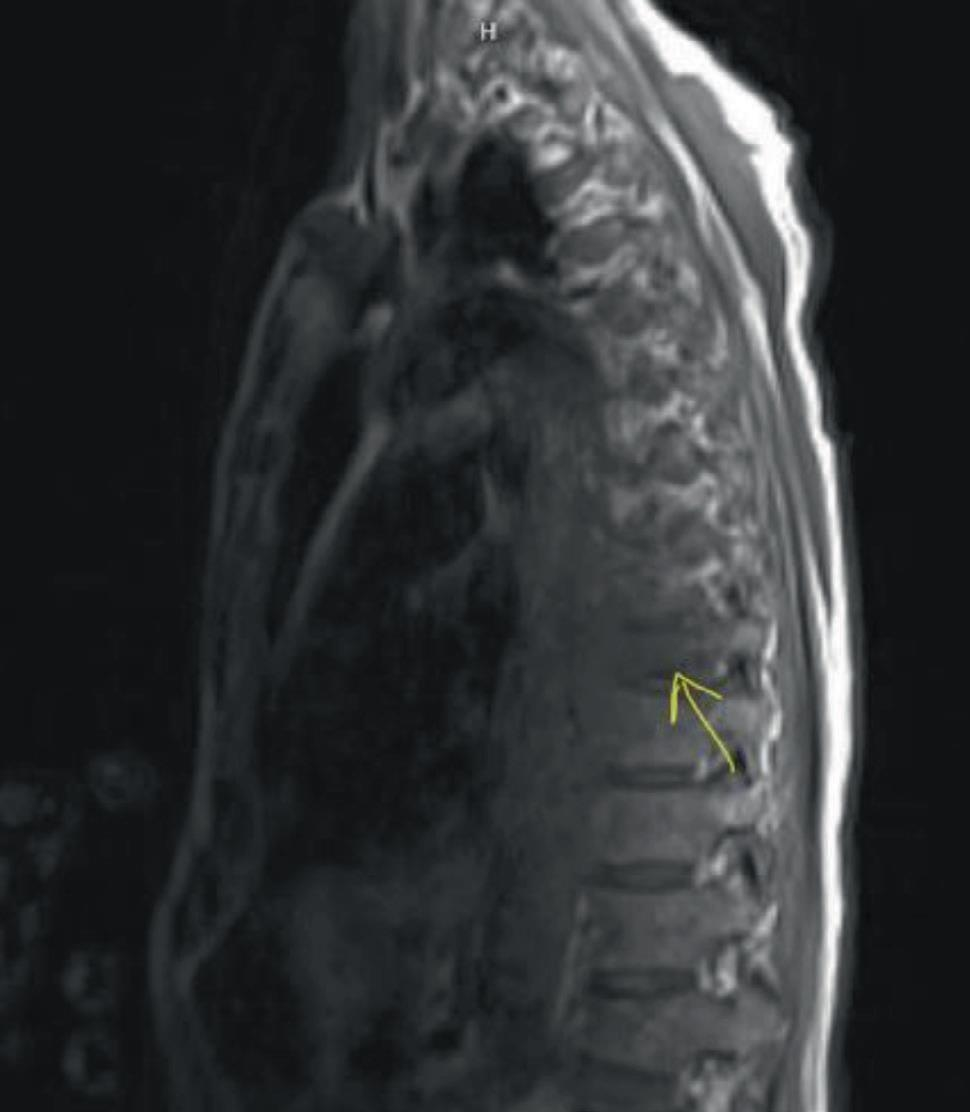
MRI showing the prevertebral mass over T9.
